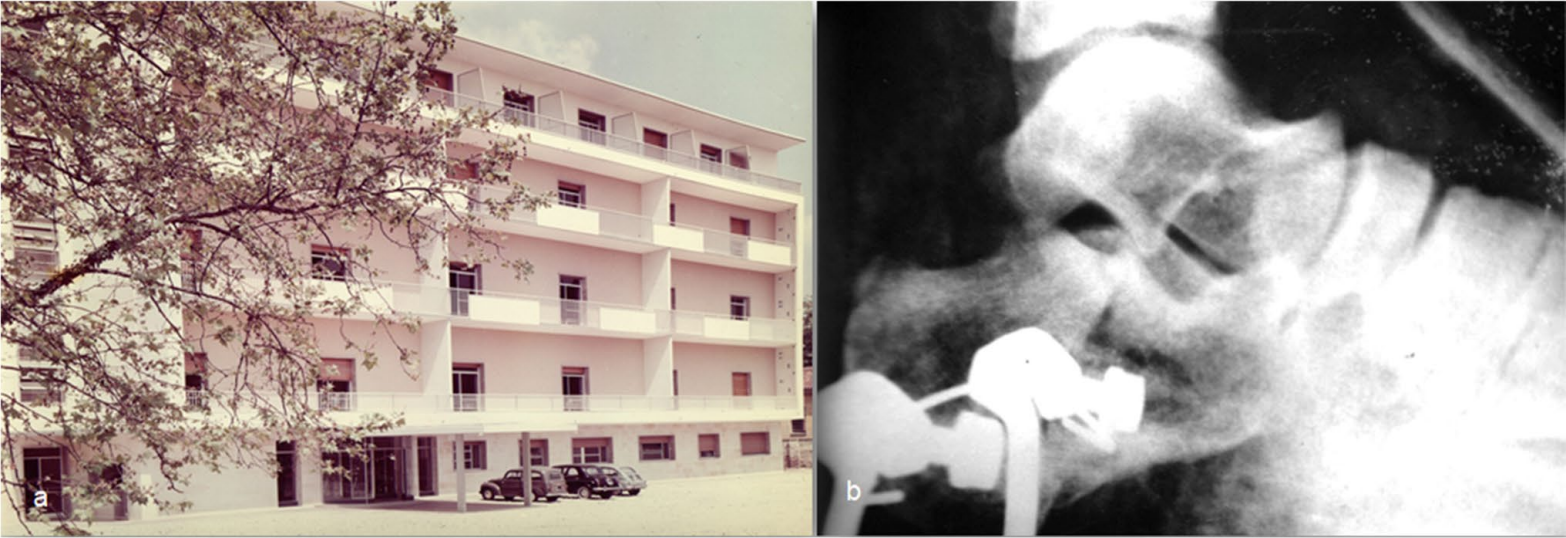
a Historical photo of the Orthopaedic Department of Padua 1959. b Intraoperative X-ray of a displaced multi-fragmented fracture with mixed sinking: one circumscribed fragment sunken vertically, the other horizontally treated with reduction by a trans-calcaneal wire (Orthopaedic Department of Padua, 1959)
